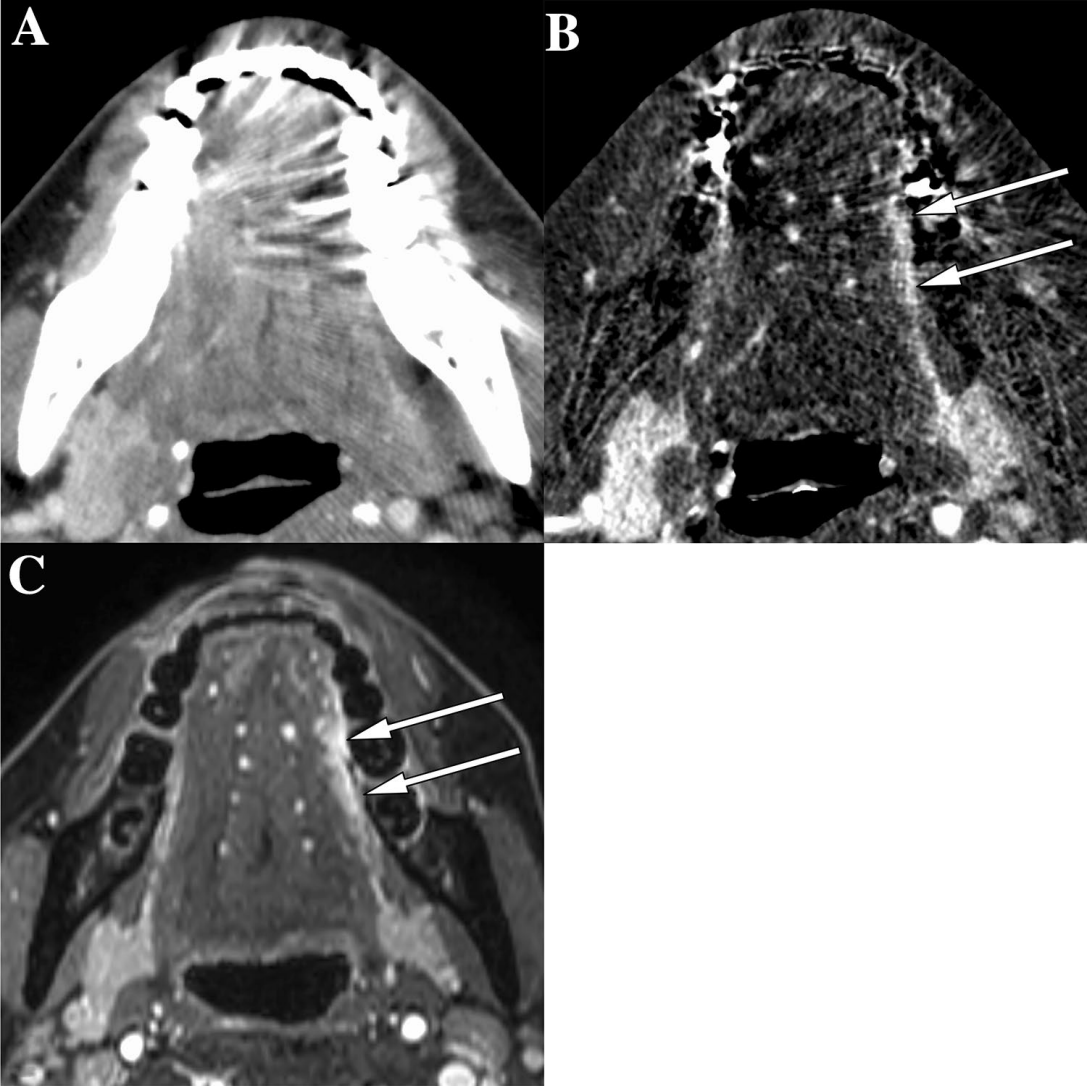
Images of a 48-year-old woman with squamous cell carcinoma of the left border of the tongue. A On CT with single-energy projection-based metallic artifact reduction algorithm, tongue cancer was not detectable due to dental artifacts. B Subtraction iodine imaging (SII) shows tumor enhancement of the left border of the tongue (white arrows in B) mainly due to reduced artifacts using subtraction. C On contrast-enhanced T1-weighted MR images with fat suppression, the tumor is detectable and measurable (white arrows in C) as well as with SII
